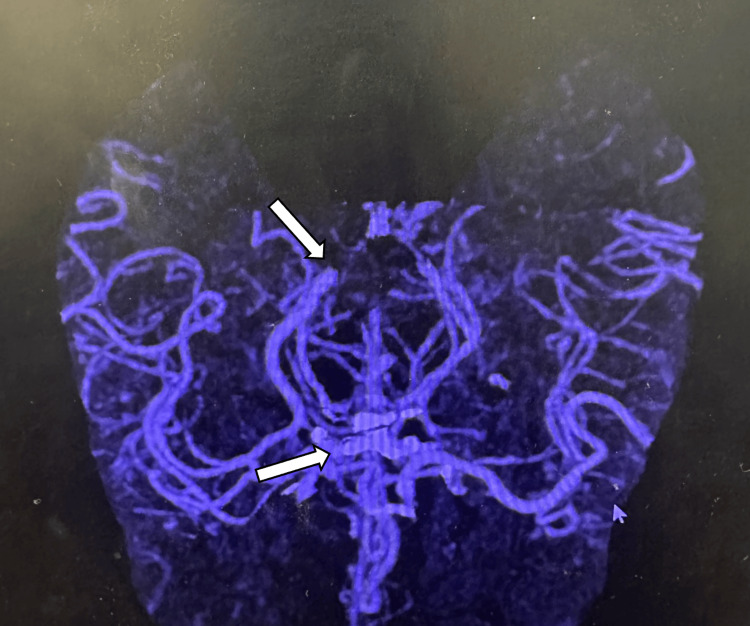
CT angiography demonstrating posterior circulation vascular anatomy. CT angiography of the head and neck demonstrates the vertebrobasilar circulation, highlighting high-grade stenosis of the basilar artery (arrow), consistent with the vascular findings described in the diagnostic evaluation.
